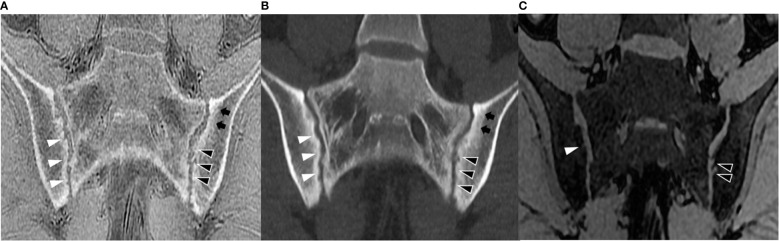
Semi-coronal images in a 45-year-old man with sacroiliitis. (A) ZTE, (B) ldCT, and (C) LAVA-Flex. Multiple erosions on the right sacroiliac joint (white arrowhead) and the left sacroiliac joint (black arrowhead) were graded as third-degree on ZTE and ldCT but graded as first-degree on the right and second-degree on the left on LAVA-Flex; both ZTE and ldCT could accurately exhibit sclerosis (small black arrow), but such sclerosis could be easily confused with fat deposition. ldCT, low-dose CT; ZTE, Zero echo time; LAVA-Flex, Liver Acquisition with Volume Acceleration-flexible.
